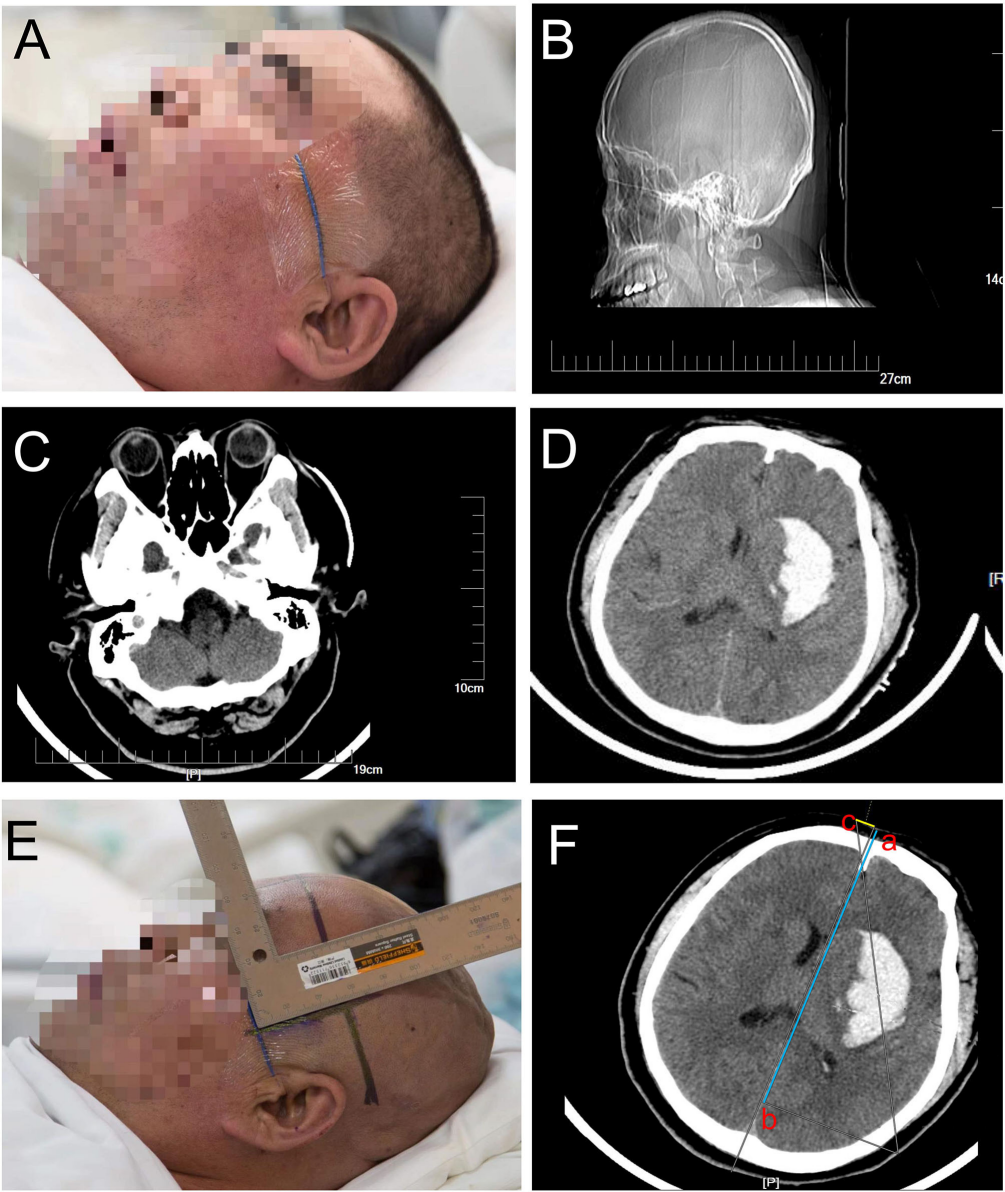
(A) shows that the orbitomeatal line is the preparation before the CT scan for patients with suspected cerebral hemorrhage, (B) confirms that the orbitomeatal line was precisely attached to the scalp, (C) represents the slice marked by the orbitomeatal line as the first slice of the CT scan, intracranial hemorrhage was confirmed in (D), and the punctured plane was measured based on the distance between the first slice and the largest slice of the hematoma in (E). (F) represents how to determine the position of the location stickers on the CT image by measuring and marking their positions on the scalp.
